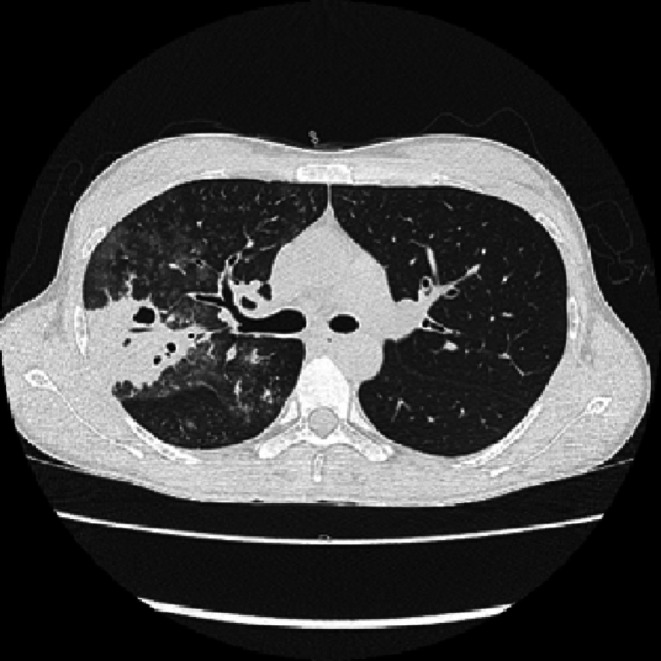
CT revealed consolidation, ground‐glass opacities, and cavitary lesions predominantly in the right upper lobe.
